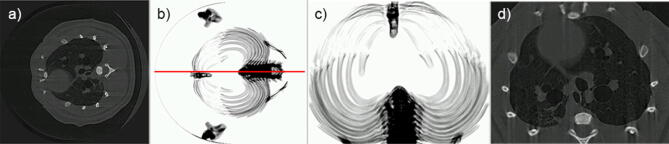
Mirror symmetry algorithm for automatic orientation and cropping. (a) A single slice from a CT reconstruction, pre-cropping. In (b), slices of the CT have been projected in the cranial/caudal direction to create a single image and the image thresholded to show the bones, revealing the symmetry of the ribcage. The red line indicates the line-of-best-symmetry as found by the algorithm, passing through the spine and sternum, allowing us to register their positions. (c) Using this result, the algorithm automatically corrects the orientation of the sample, and crops the image to the bones that act as a boundary for lung tissue; (d) shows a rotated and cropped slice*.*
